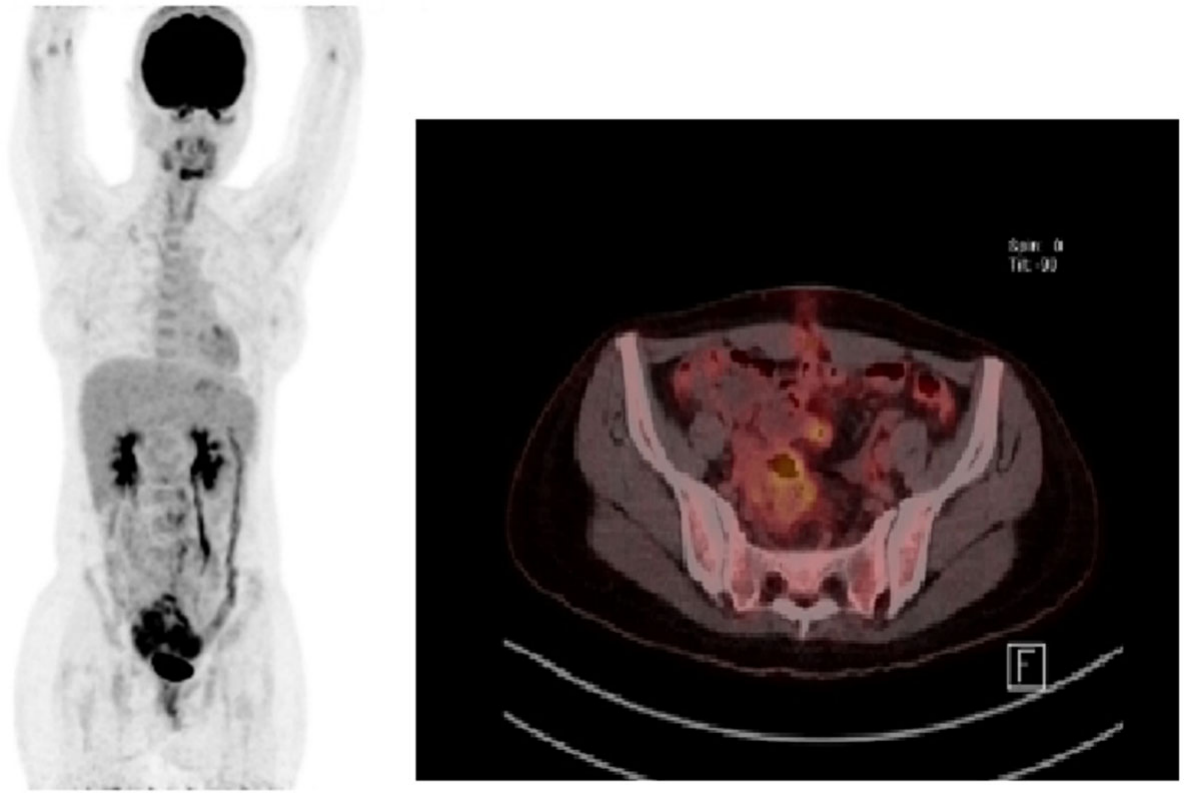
FDG-PET scan show no metastatic lesions in other organ
